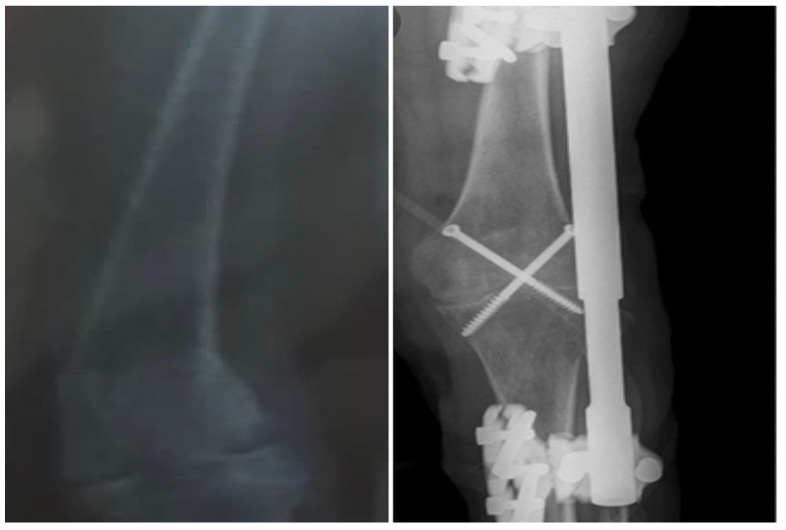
Plain radiograph of the left knee with lateral locking plate after the insertion of new cross-pins following a full recovery from osteomyelitis.
